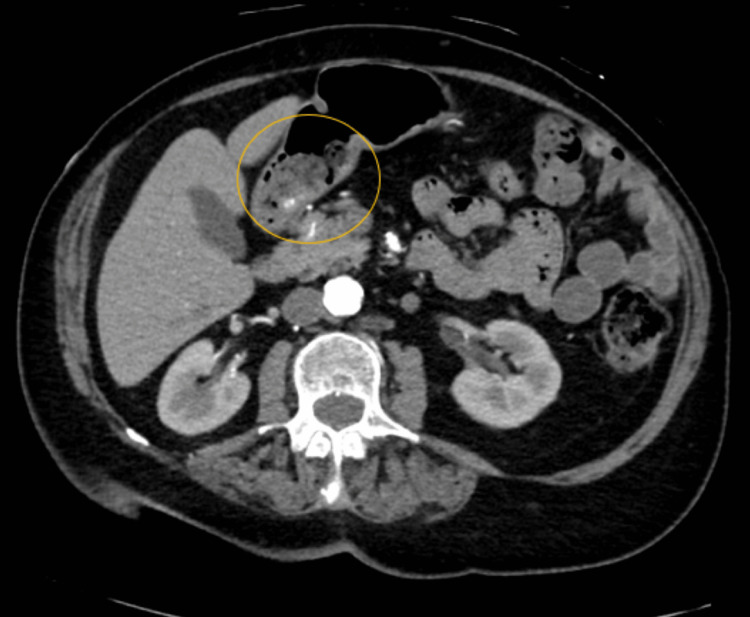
CTA of the abdomen: questionable small focus of hemorrhage in the posterior gastric antrum. CTA: computed tomography-angiography
